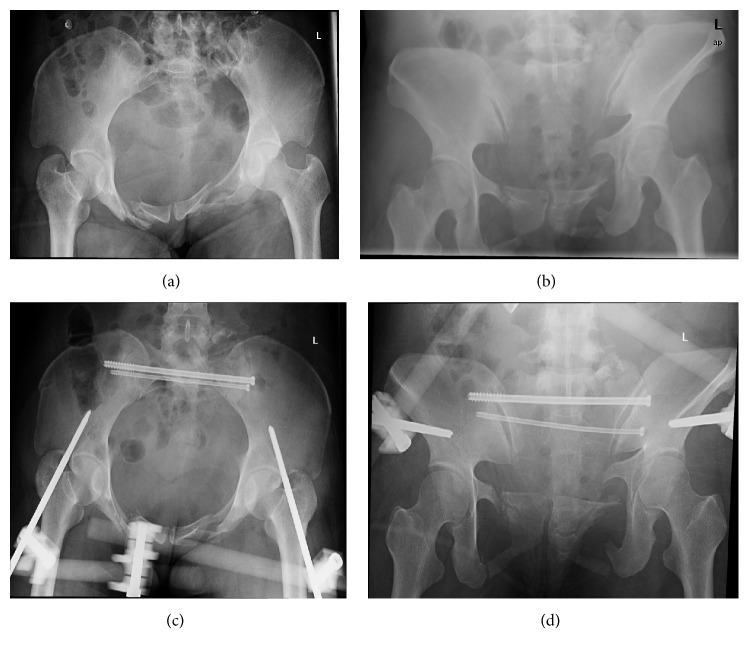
Case number 2. (a) and (b) Preoperative inlet and outlet view radiographs; (c) and (d) postoperative inlet and outlet view radiographs.
